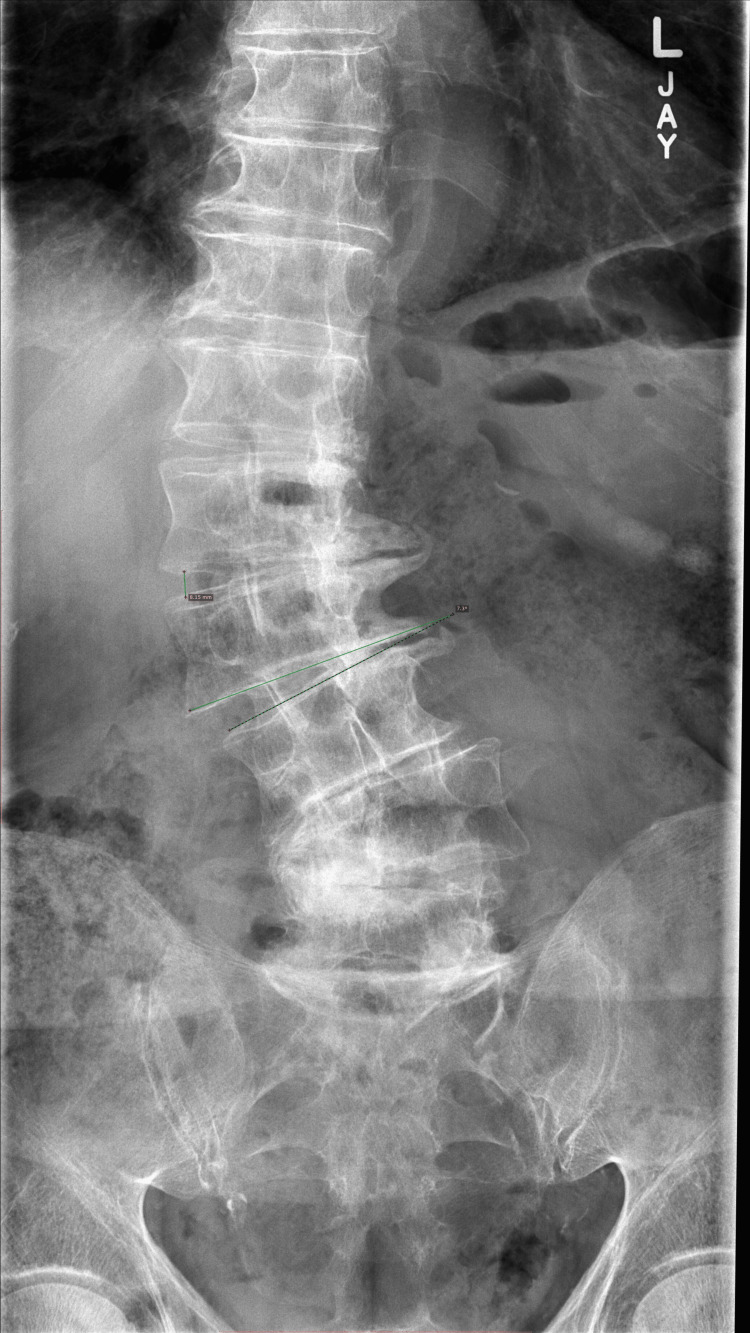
Spinal stenosis, L4-L5 disk bulge, neuroforaminal narrowing This radiograph shows spinal stenosis at the L4-L5 level, combined with disk bulge and neuroforaminal narrowing. Provider assessment of the condition indicated nerve openings (foramina) had become constricted as outlined in image. Age-related wear and disk damage have been cited in the literature as examples of nerve compression and symptoms like leg pain, numbness, tingling, and weakness.
